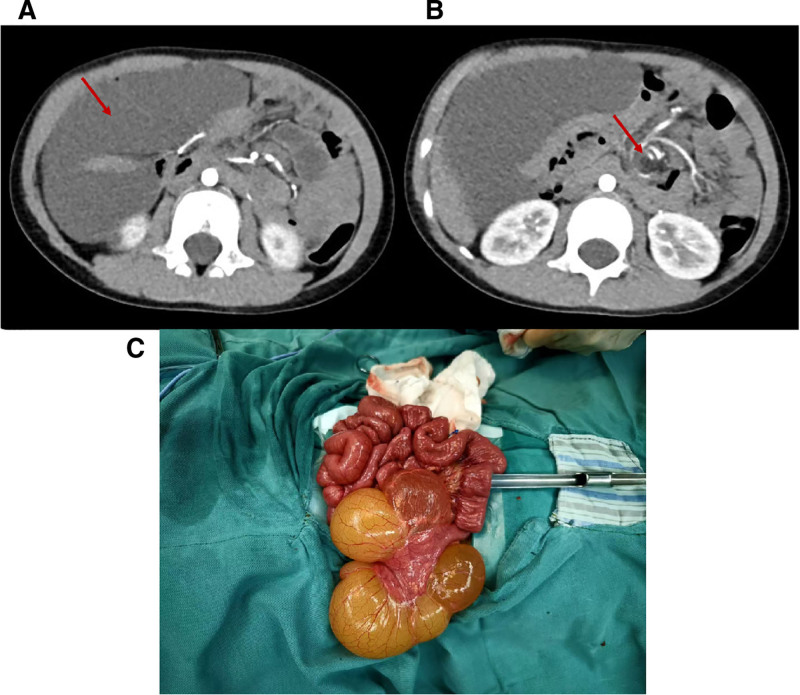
A patient with mesenteric lymphangioma accompanied by SBV. (A) A contrast-enhanced abdominal CT scan revealed a cystic, space-occupying lesion. (B) The CT image demonstrated a whirlpool sign, indicative of the superior mesenteric vein wrapping around the superior mesenteric artery. (C) Post-devolvulation, the lesion connected to the mesentery was excised, with resection performed on the anal side, 30 cm distal to the Treitz ligament. CT = computed tomography, SBV = small-bowel volvulus.
